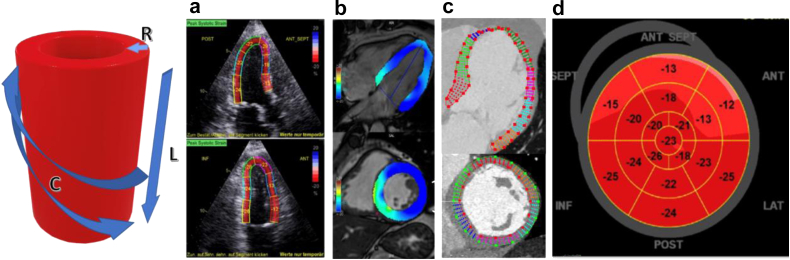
Cardiac deformation imaging with different modalities in an example of the left ventricle. The graphic on the left picturizes the directions of myocardial shortening within the different layers of the myocardium. The endocardial layer is formed by fibers with longitudinal (L) orientation. The mid-wall has a radial (R) orientation, while the epicardium is circumferentially (C) orientated. Using different imaging modalities, the shortening along the three directions can be visualized. The calculations are based on the tracking of individual pixels within a cine projection of the cardiac cycle. This method can be performed in a similar way for the other chambers of the heart. (a) Echo-strain imaging (Uhe et al. 2020,^53^ © CC-BY 4.0); (b) CMR-feature tracking (Scatteia et al. 2017,^54^ © CC-BY 4.0) and (c) CT-strain imaging (Vach et al. 2021,^55^ © CC-BY 4.0). (d) shows an example of a Bulls‘ eye trajectory of the myocardium with the relative shortening (%) for each region of the myocardium (Uhe et al. 2020,^53^ © CC-BY 4.0). https://creativecommons.org/licenses/by/4.0/.
